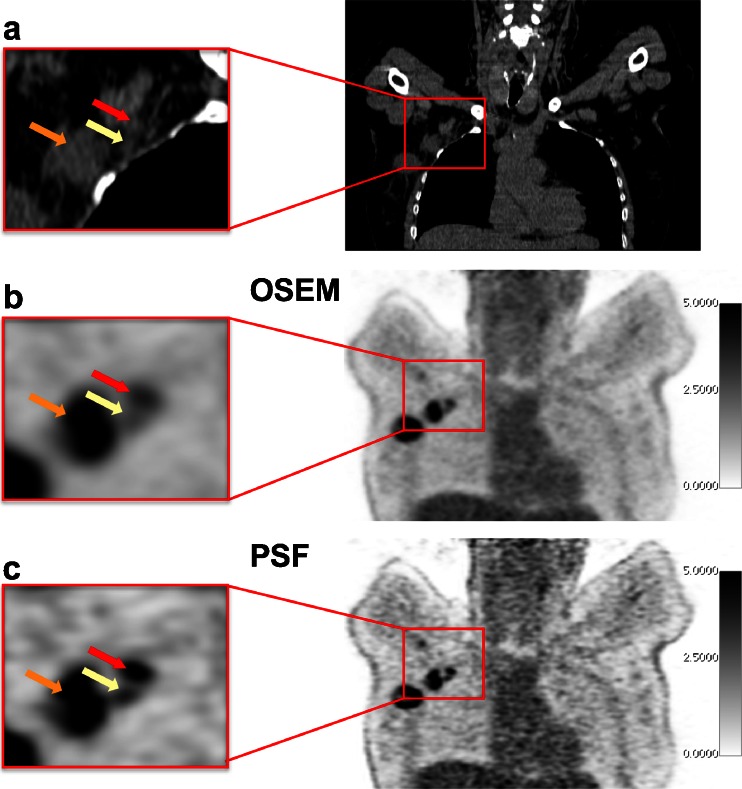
A 51-year-old patient with grade III infiltrating ductal carcinoma initially classified as T3N0c, in whom both PSF reconstruction and OSEM reconstruction were positive, but PSF reconstruction depicted an additional involved lymph node (*yellow arrows*) (a CT images, b PSF reconstruction, c OSEM reconstruction). Both OSEM and PSF reconstructions are scaled to the same maximum value. Note how PSF reconstruction improves activity recovery in small lesions (*red and yellow arrows*) as compared with the largest node (*orange arrows*)
